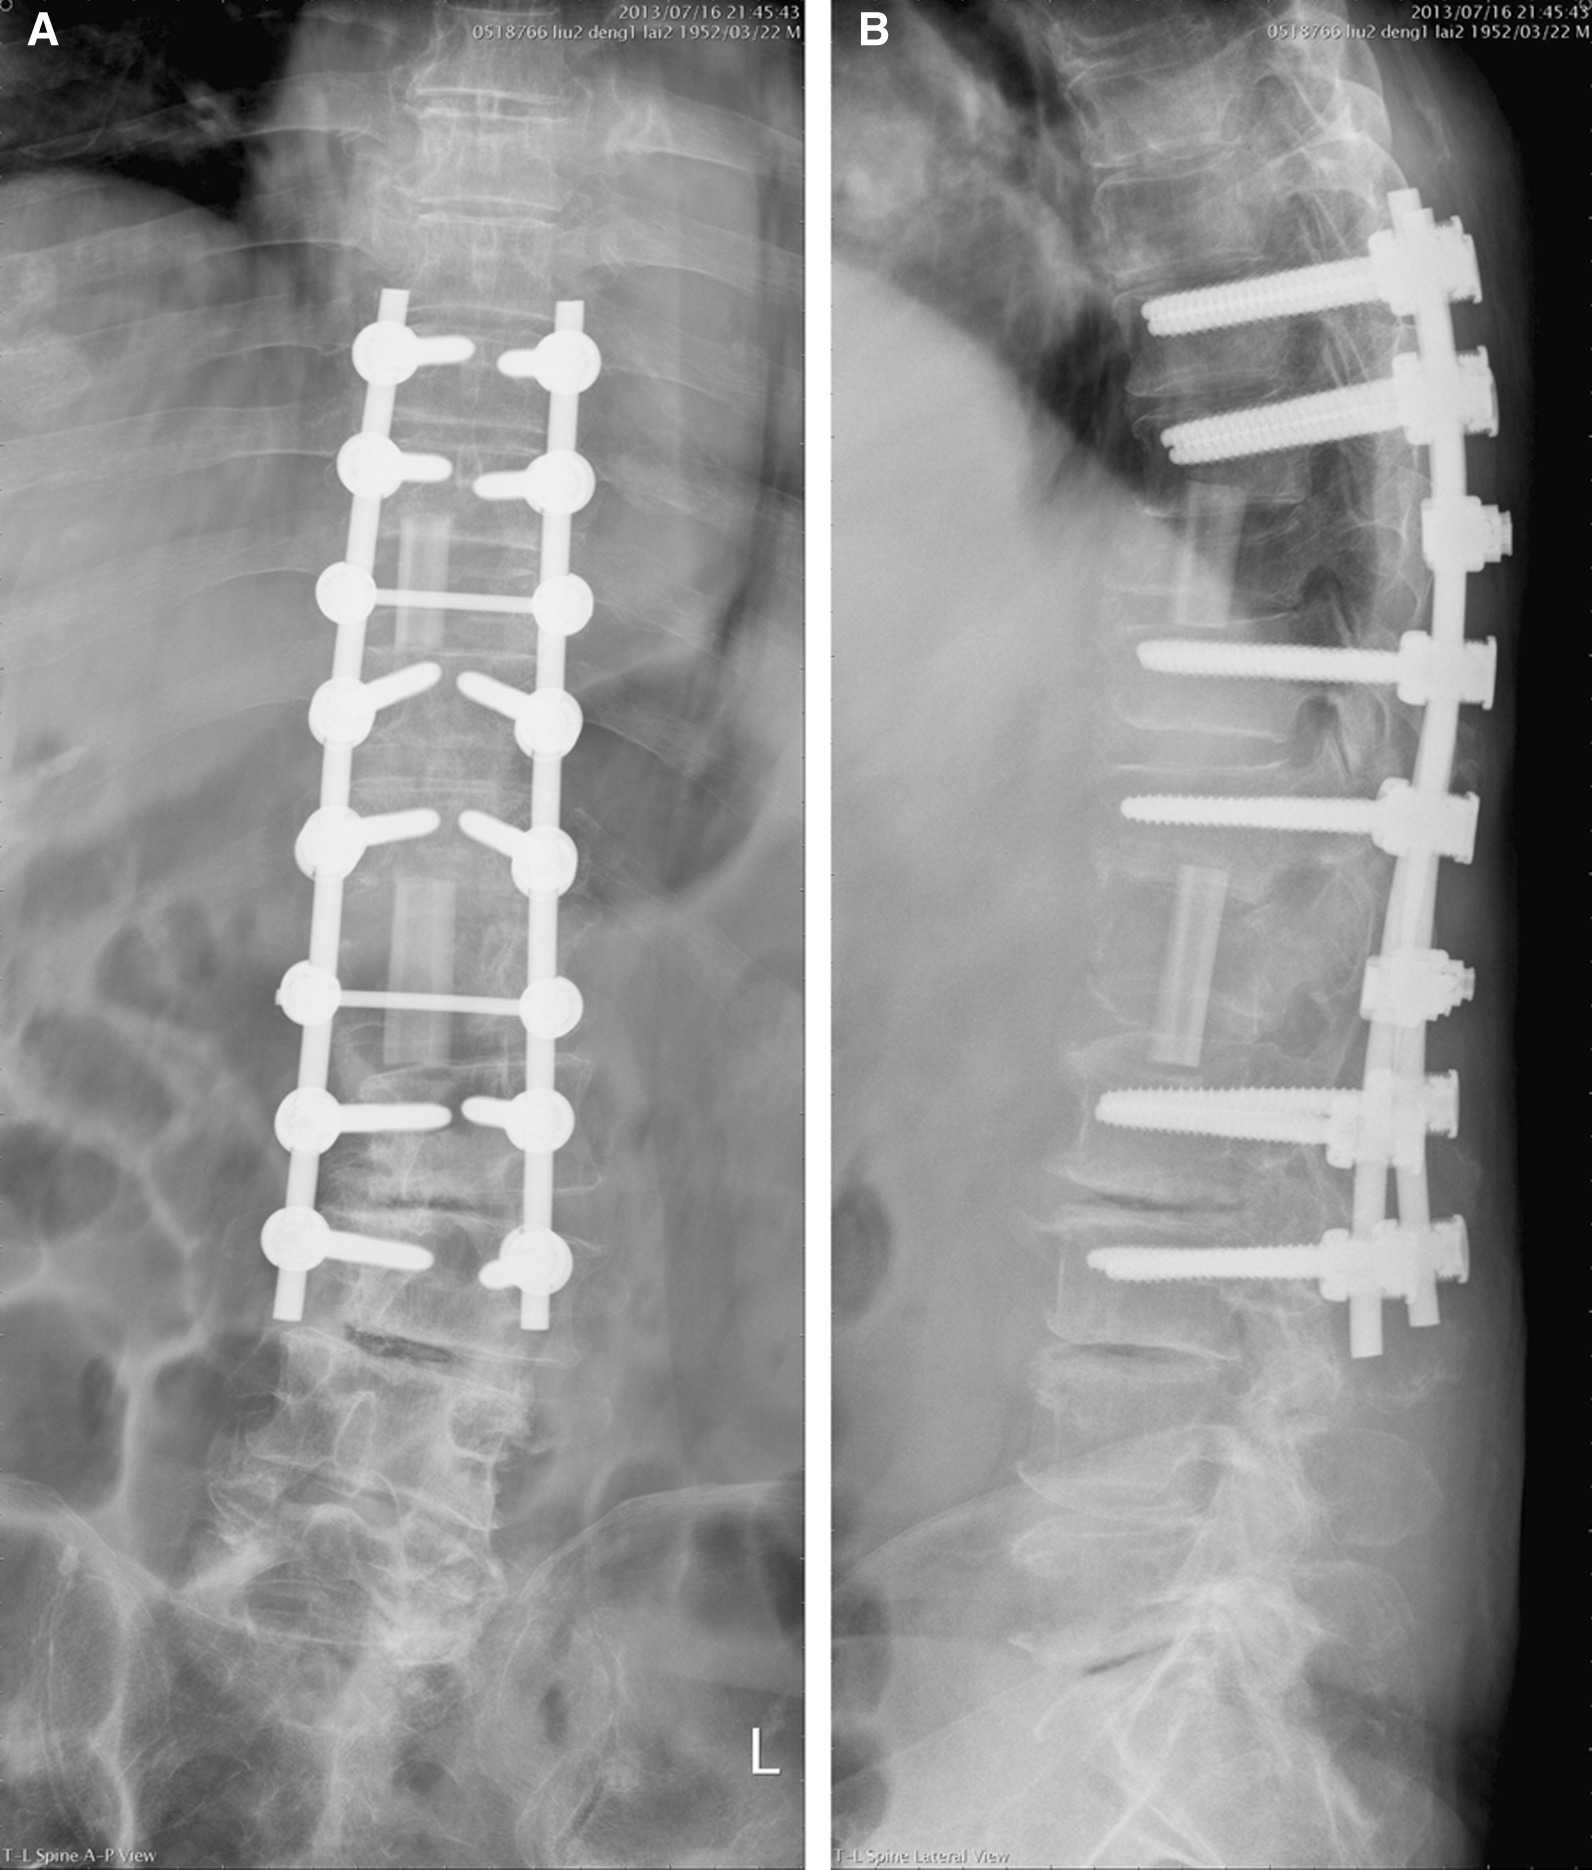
The postoperative anteroposterior (A) and lateral (B) radiographs reveal an acceptable alignment, which was restored with adequate length of allogenic fibular bone grafts and posterior instrumentation
